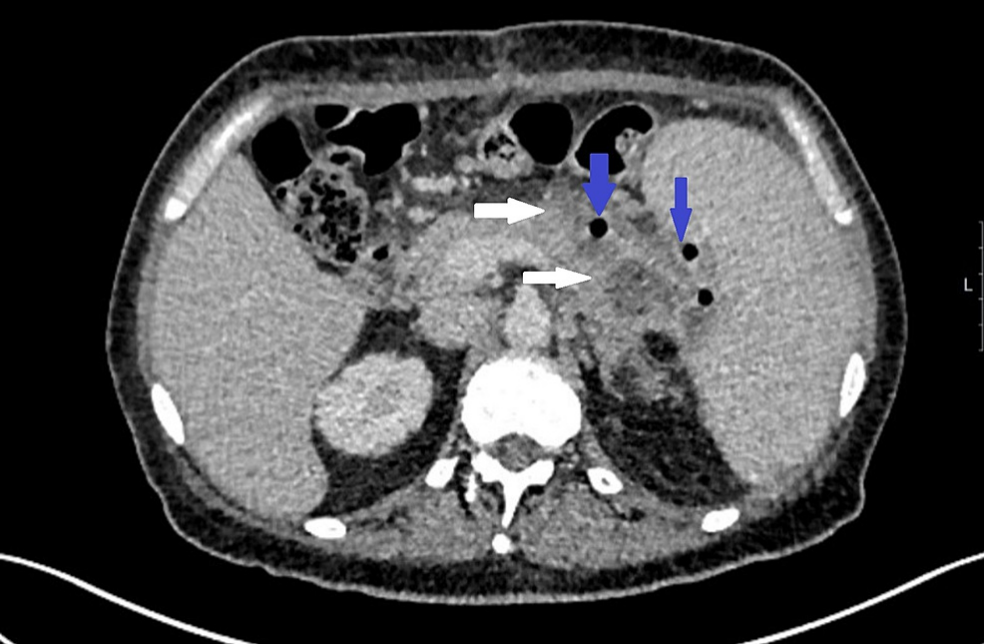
Axial CT scan showing acute necrotising pancreatitis. See non-enhancing low attenuation areas (white arrows) and extraluminal gas bubbles (blue arrows).
